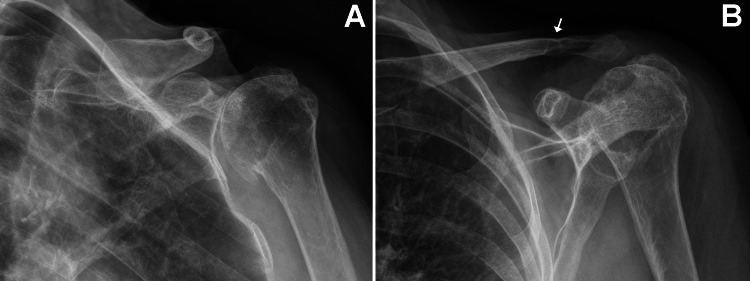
Preoperative radiographs of the patient’s left shoulder showing end-stage rotator cuff-tear arthropathy (A) Anterior-posterior view; (B) oblique scapula Y view. The site of the prior clavicle fracture has patchy lucencies (arrow) that suggest compromised bone density. Generalized osteoporosis is evident in these radiographs (reduced bone density, thin cortices).
